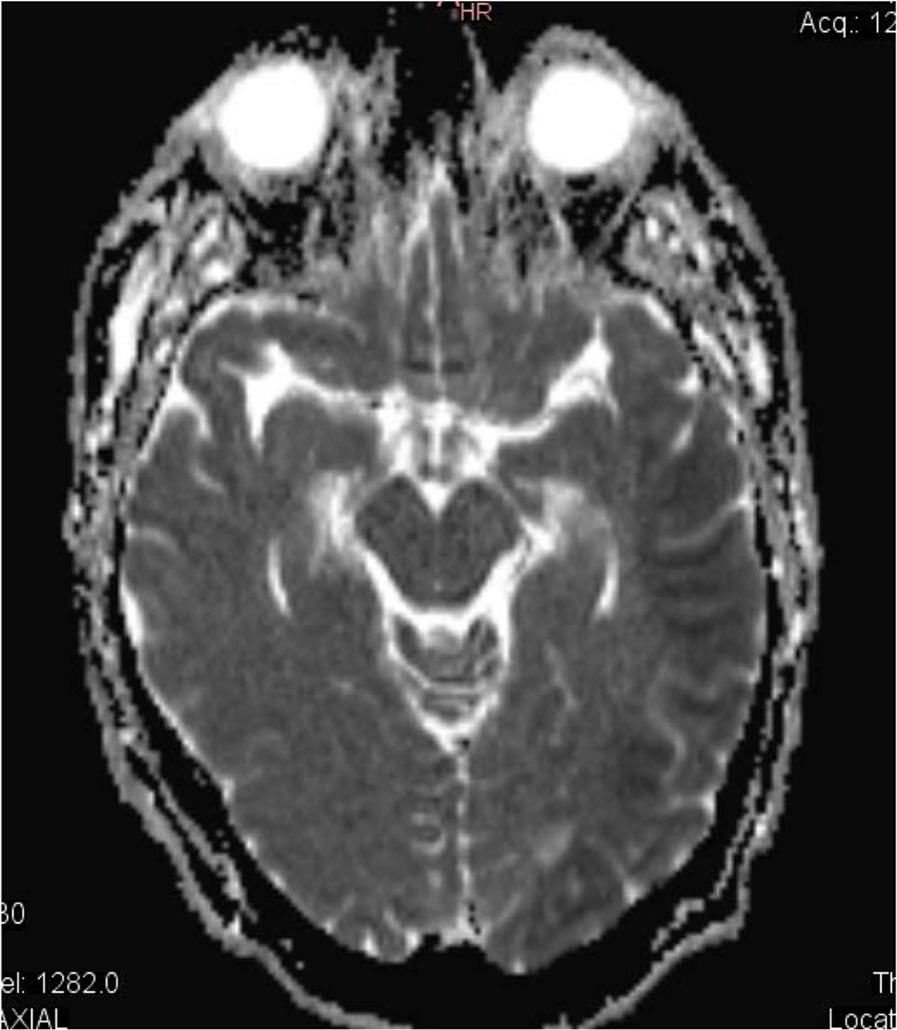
MRI diffusion sequences showing restricted diffusion in the left temporal parietal and occipital areas
